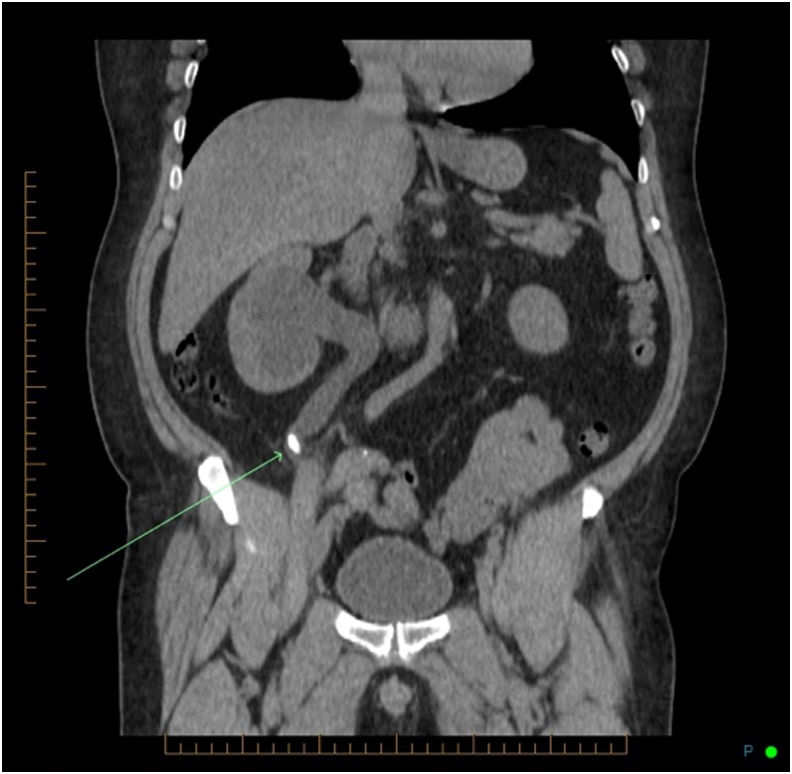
Initial coronal noncontrast CT scan image showing a 1.8 cm midureteral stone, right hydroureteronephrosis proximal to the stone, and thin right renal cortex.
